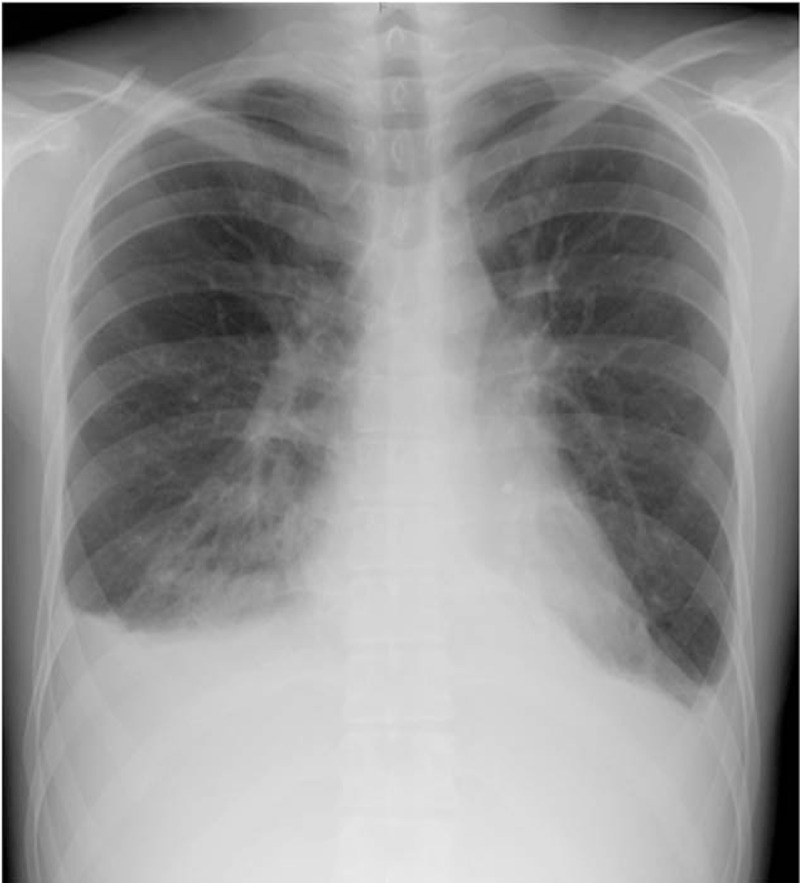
Chest X-ray performed on day 10 demonstrated the dilatation of chest-thoracic ratio and bilateral pleural effusion.
